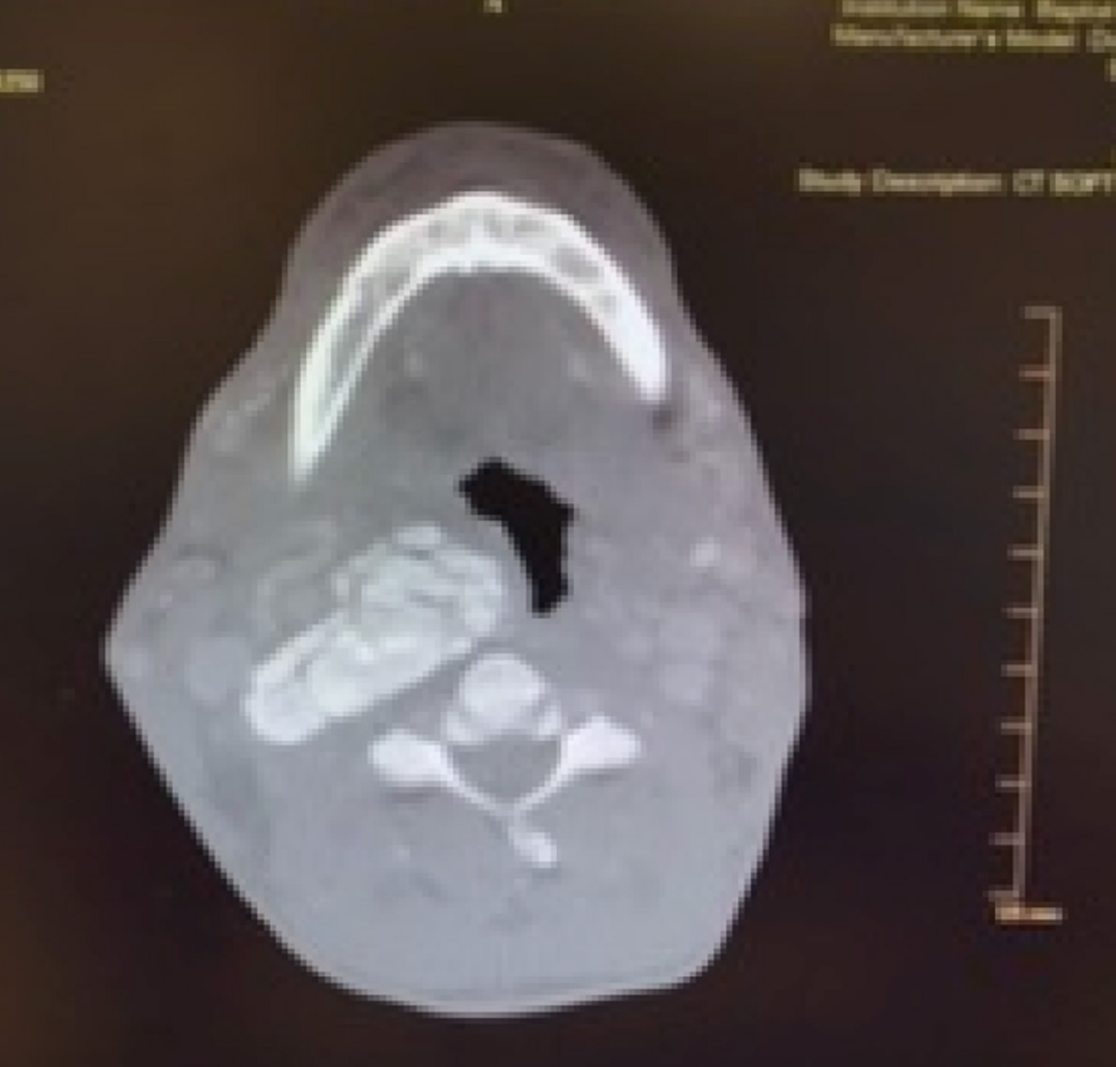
Axial view, CT soft tissue neck. Large multilobulated calcified mass
